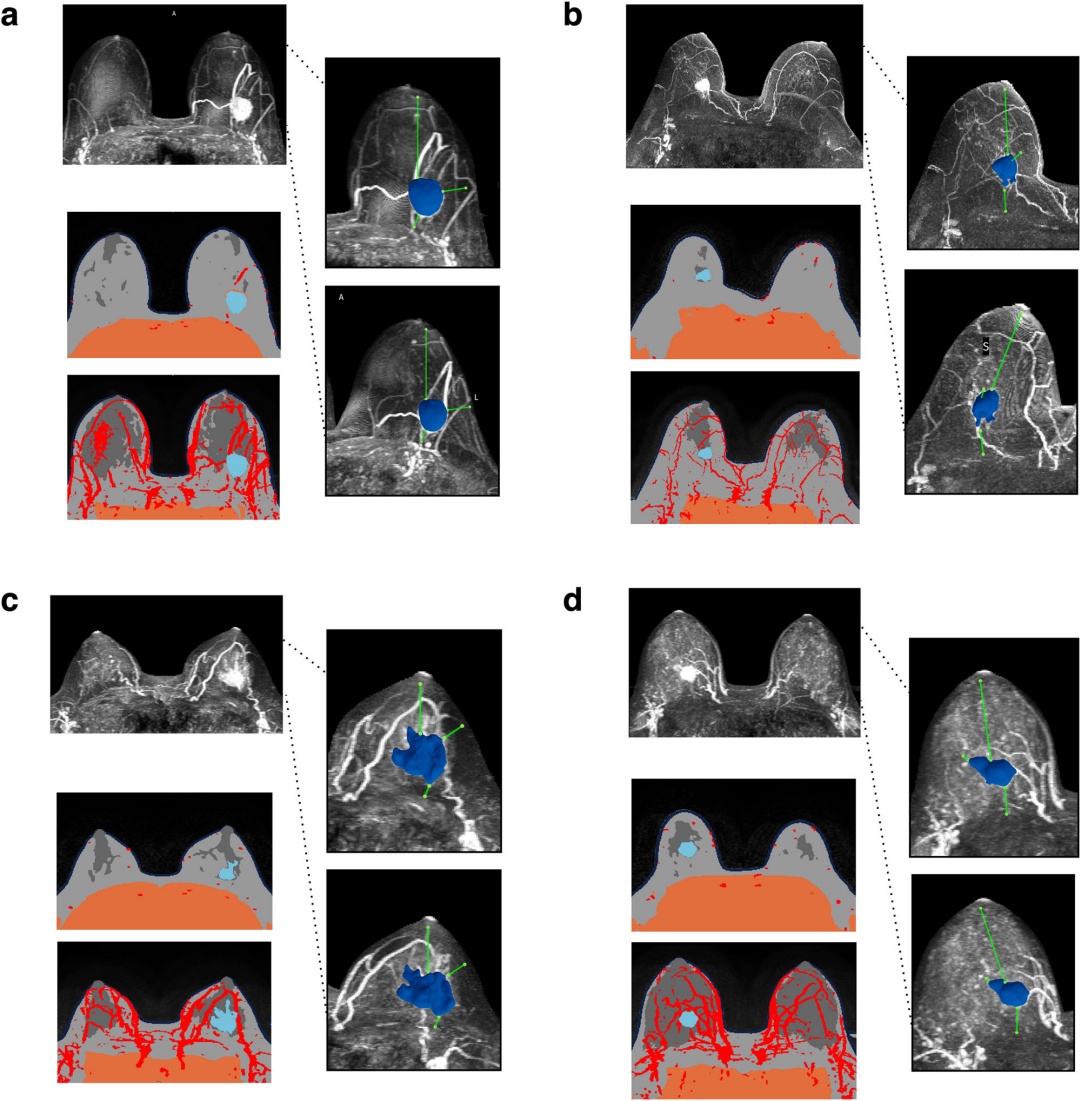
Radiologist-verified depictions of early-stage breast cancer in the UAB validation cohort, as rendered in TumorSight Viz. For each case in (a–d), the heart-masked DCE-MRI (left-top) is shown along with a single MRI slice (left-mid) as well as the maximum intensity projection (left-bottom). Renderings of TumorSight Viz segmentations from two perspectives are shown at right.
